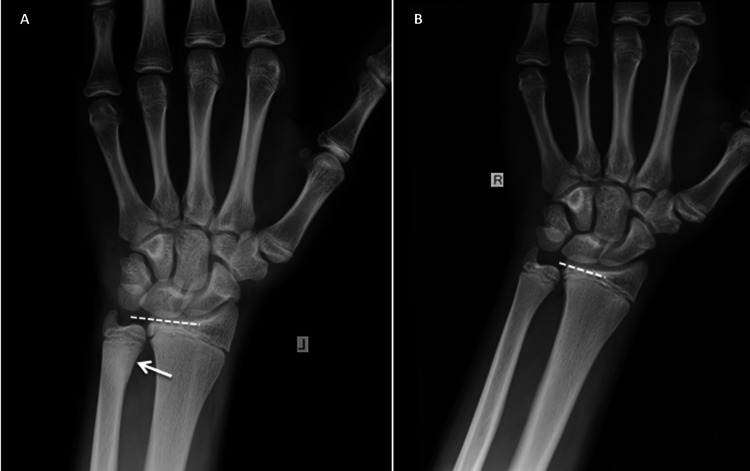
Anteroposterior radiographs of the left (A) and the right (B) wrists at six months following injury.
